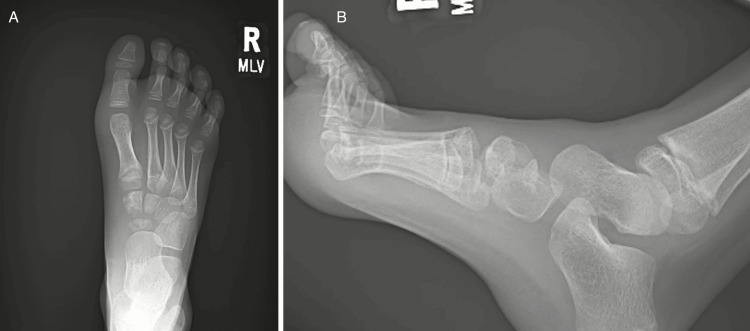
Radiographs from Patient 2’s initial visit, including anteroposterior (A) and lateral (B) views of the right foot of the eight-year-old patient. Loss of the medial margin of both the navicular and medial cuneiform can be seen.
